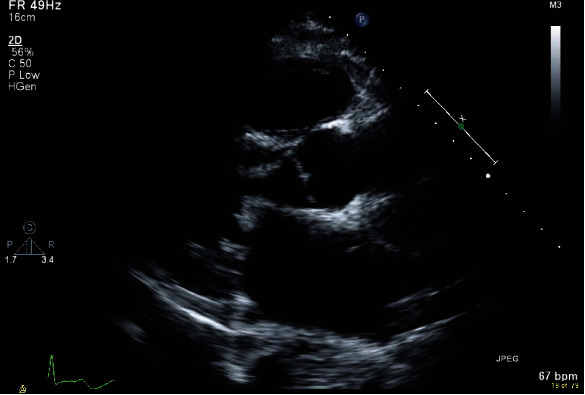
Parasternal long axis view revealing a diffused leaflet thickening of the mitral valve.
